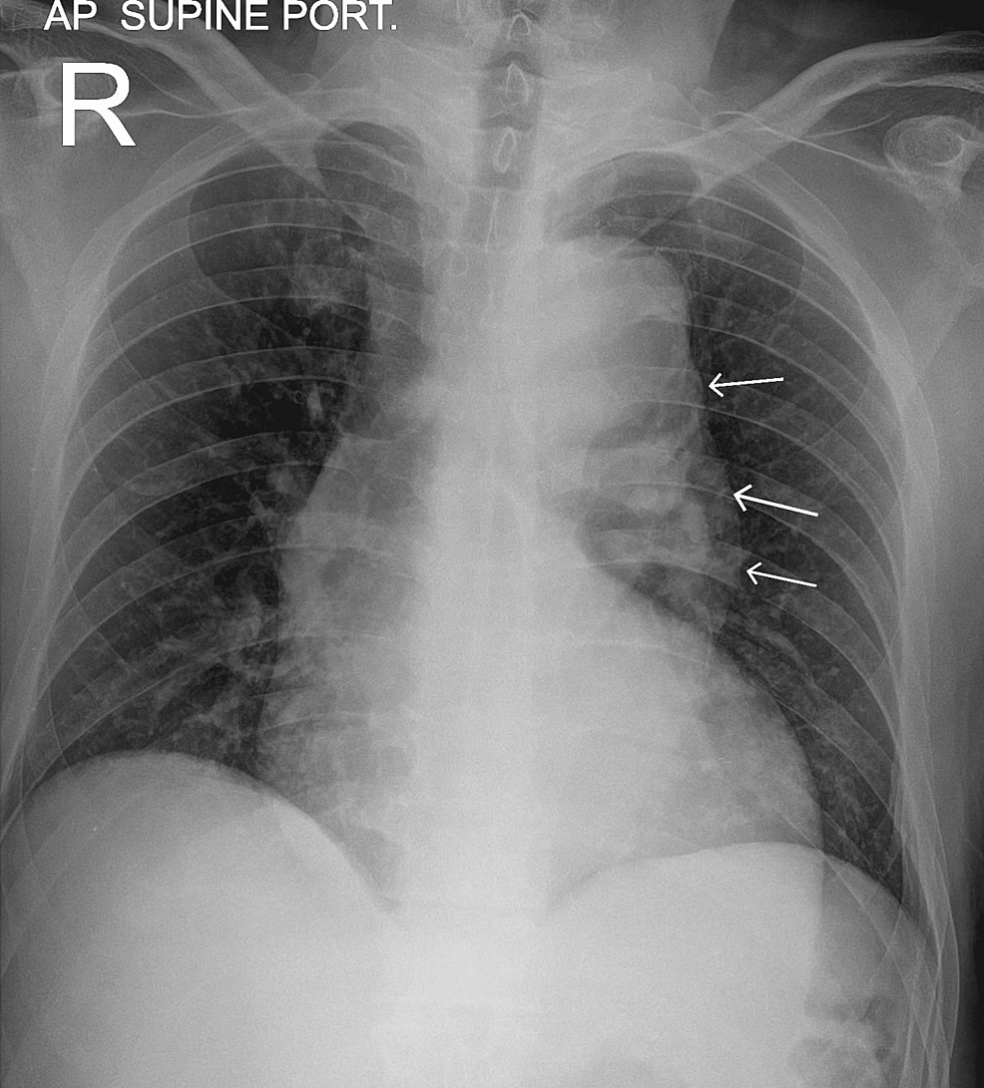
X-ray chest showing wide mediastinum (white arrows)
